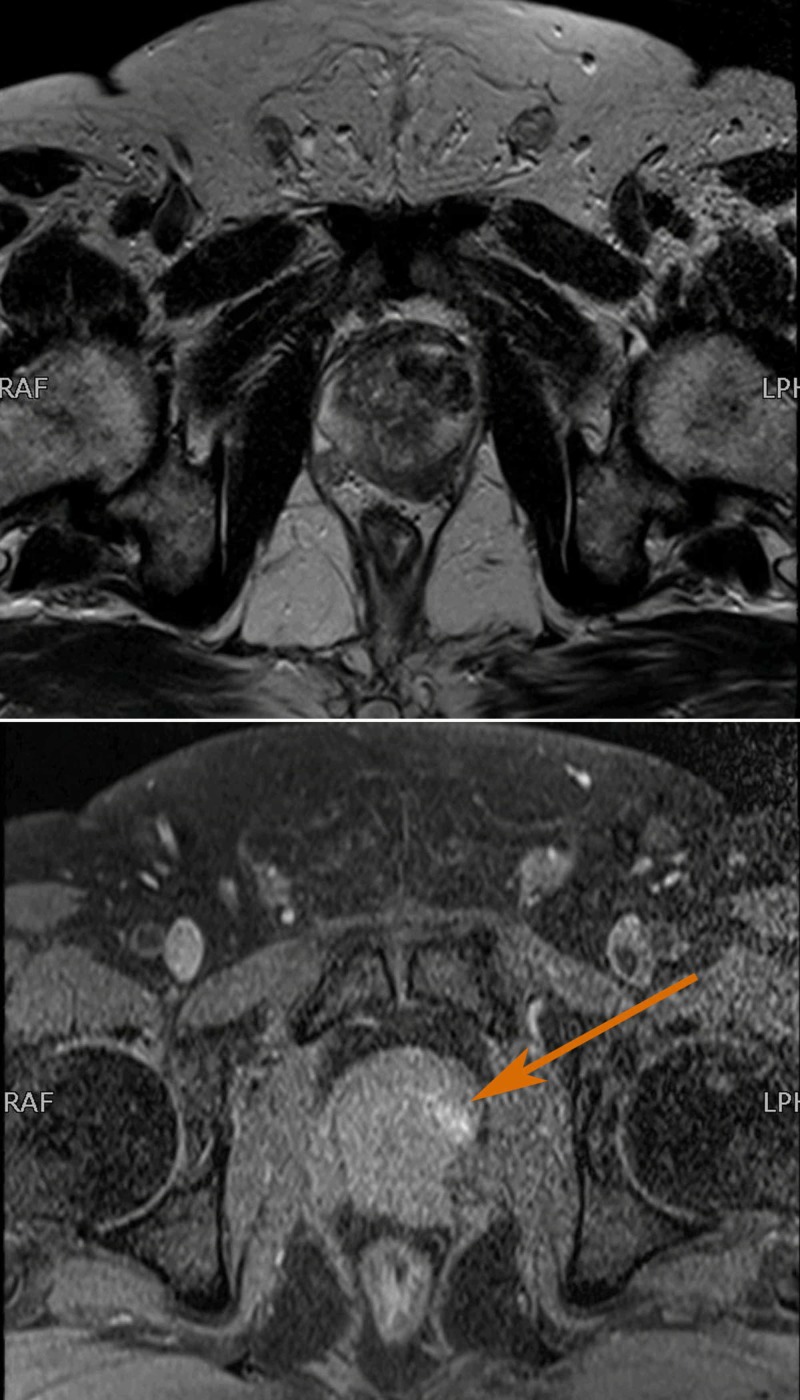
Pre-treatment MRI pelvis T1 with contrast (lower) and T2 (upper) axial images MIR showing abnormal signals identified in the left lobe of the prostate gland which predominantly involves the mid and basal zone and is crossing the midline. Posteriorly, on the left side, there is a focal breech in capsule and enhancement with central non-enhancing area suggestive of necrosis/haemorrhage.
